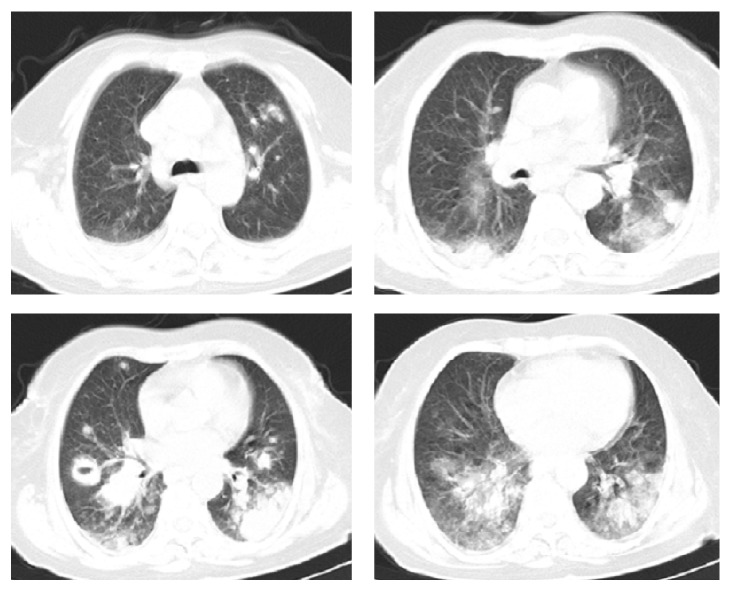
Parenchymal window of computed tomography scan showing parenchymal nodules, infiltrations, pleural masses, and cavitary lesion.
